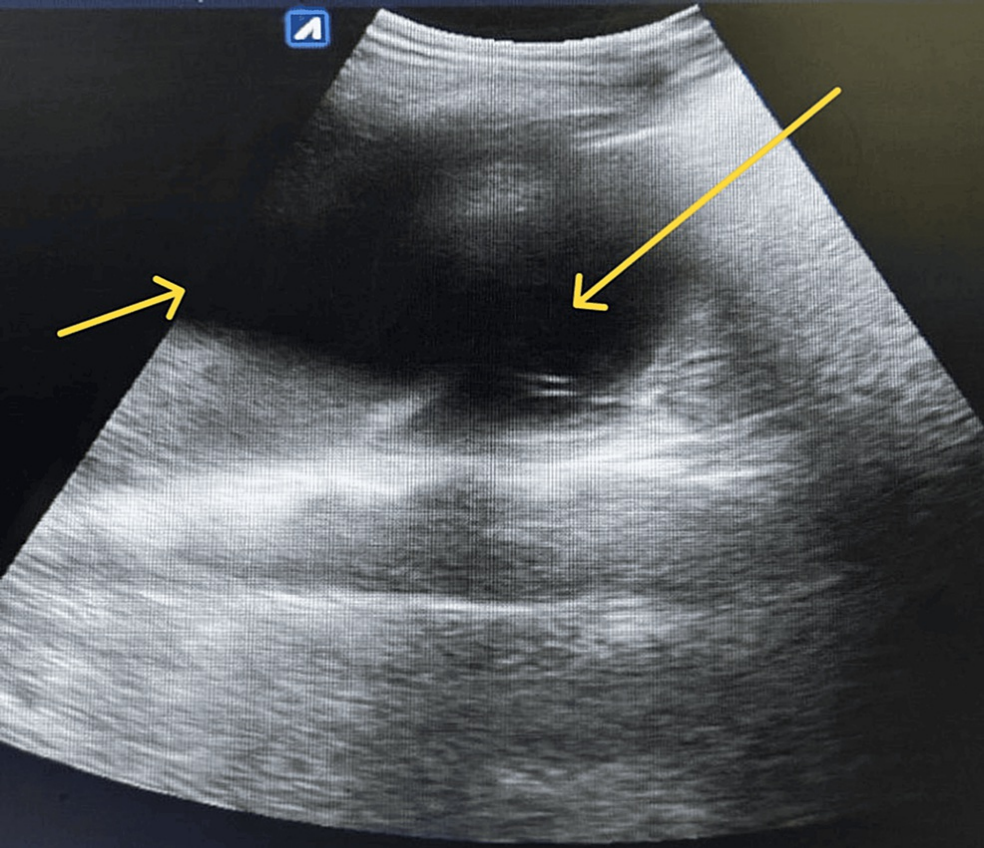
Ultrasonography of the left hemithorax showing moderate pleural effusion (yellow arrows)
